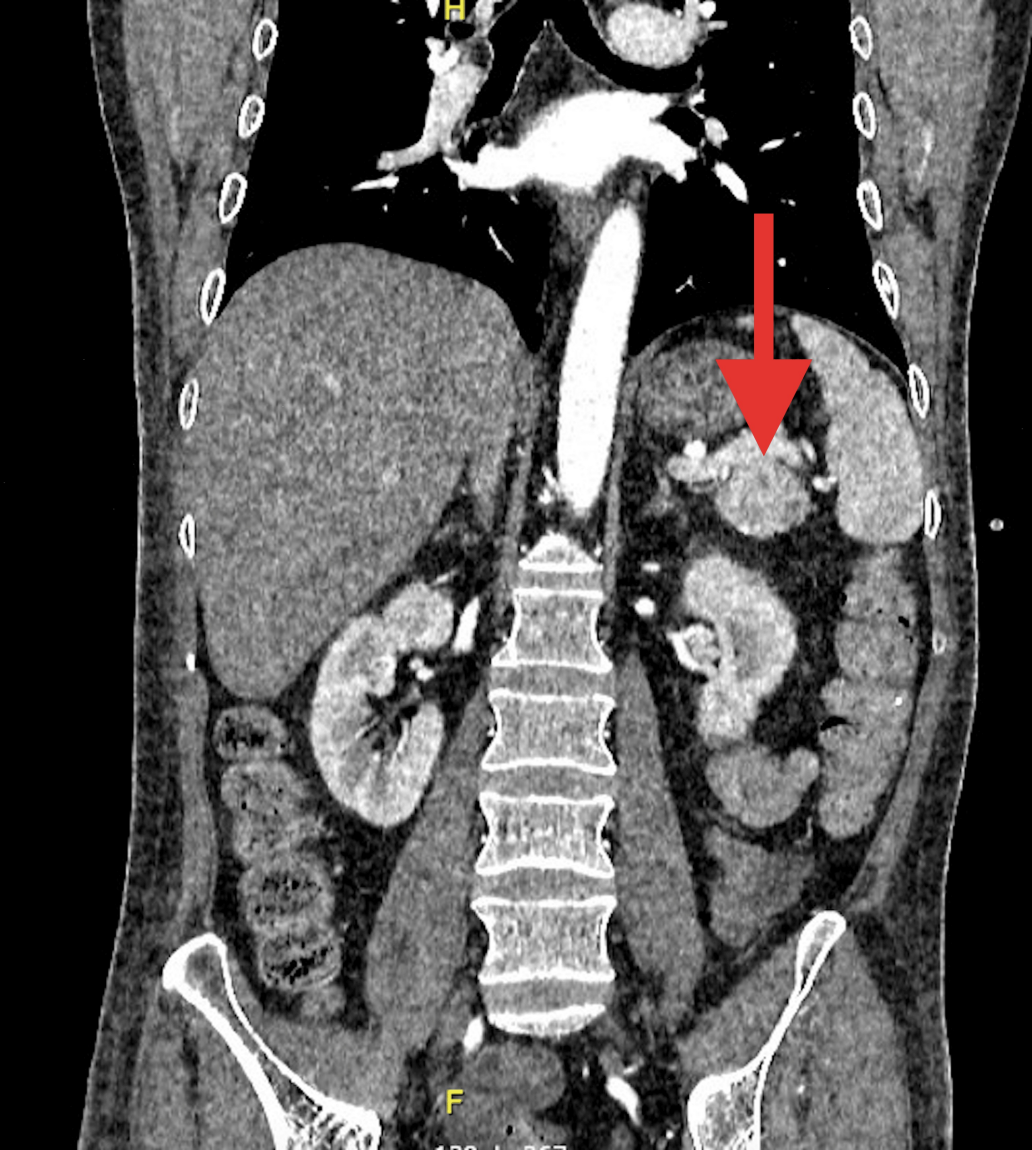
Coronal computed tomography scan highlighting a pancreatic glucagonoma This coronal CT scan depicts a well-defined mass in the tail of the pancreas, indicated by the red arrow, consistent with a glucagonoma.
